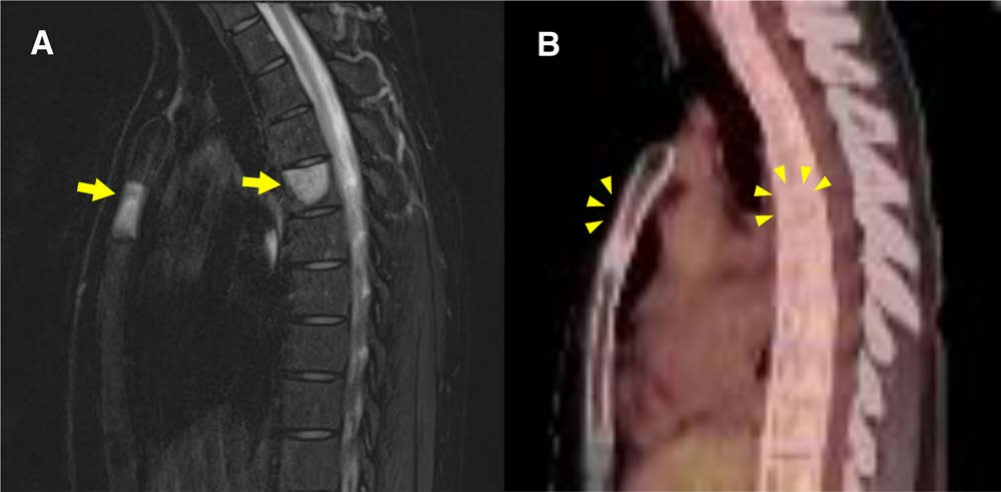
Sagittal Gd-enhanced magnetic resonance imaging (MRI) showed prolonged contrast effects in the sternum and the fifth thoracic vertebra (yellow arrows), suggesting multiple bone metastases (A). However, sagittal fluorine-18 fluorodeoxyglucose positron emission tomography/computed tomography (^18^F-FDG PET/CT) found no uptake or osteolytic destruction (yellow arrowheads), which is inconsistent with the MRI findings (B).
